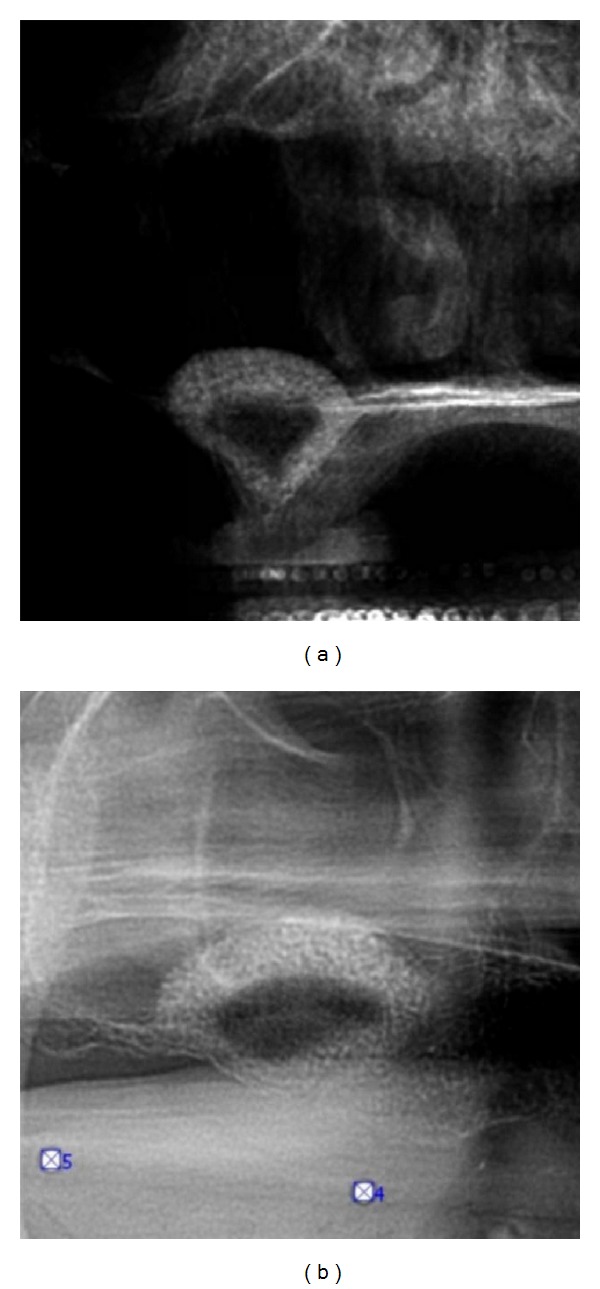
Transversal and parasagittal CBCT scan 4 months subsequent to tHUCSL-Intralift. The even circular centripetal calcification process can be observed.
